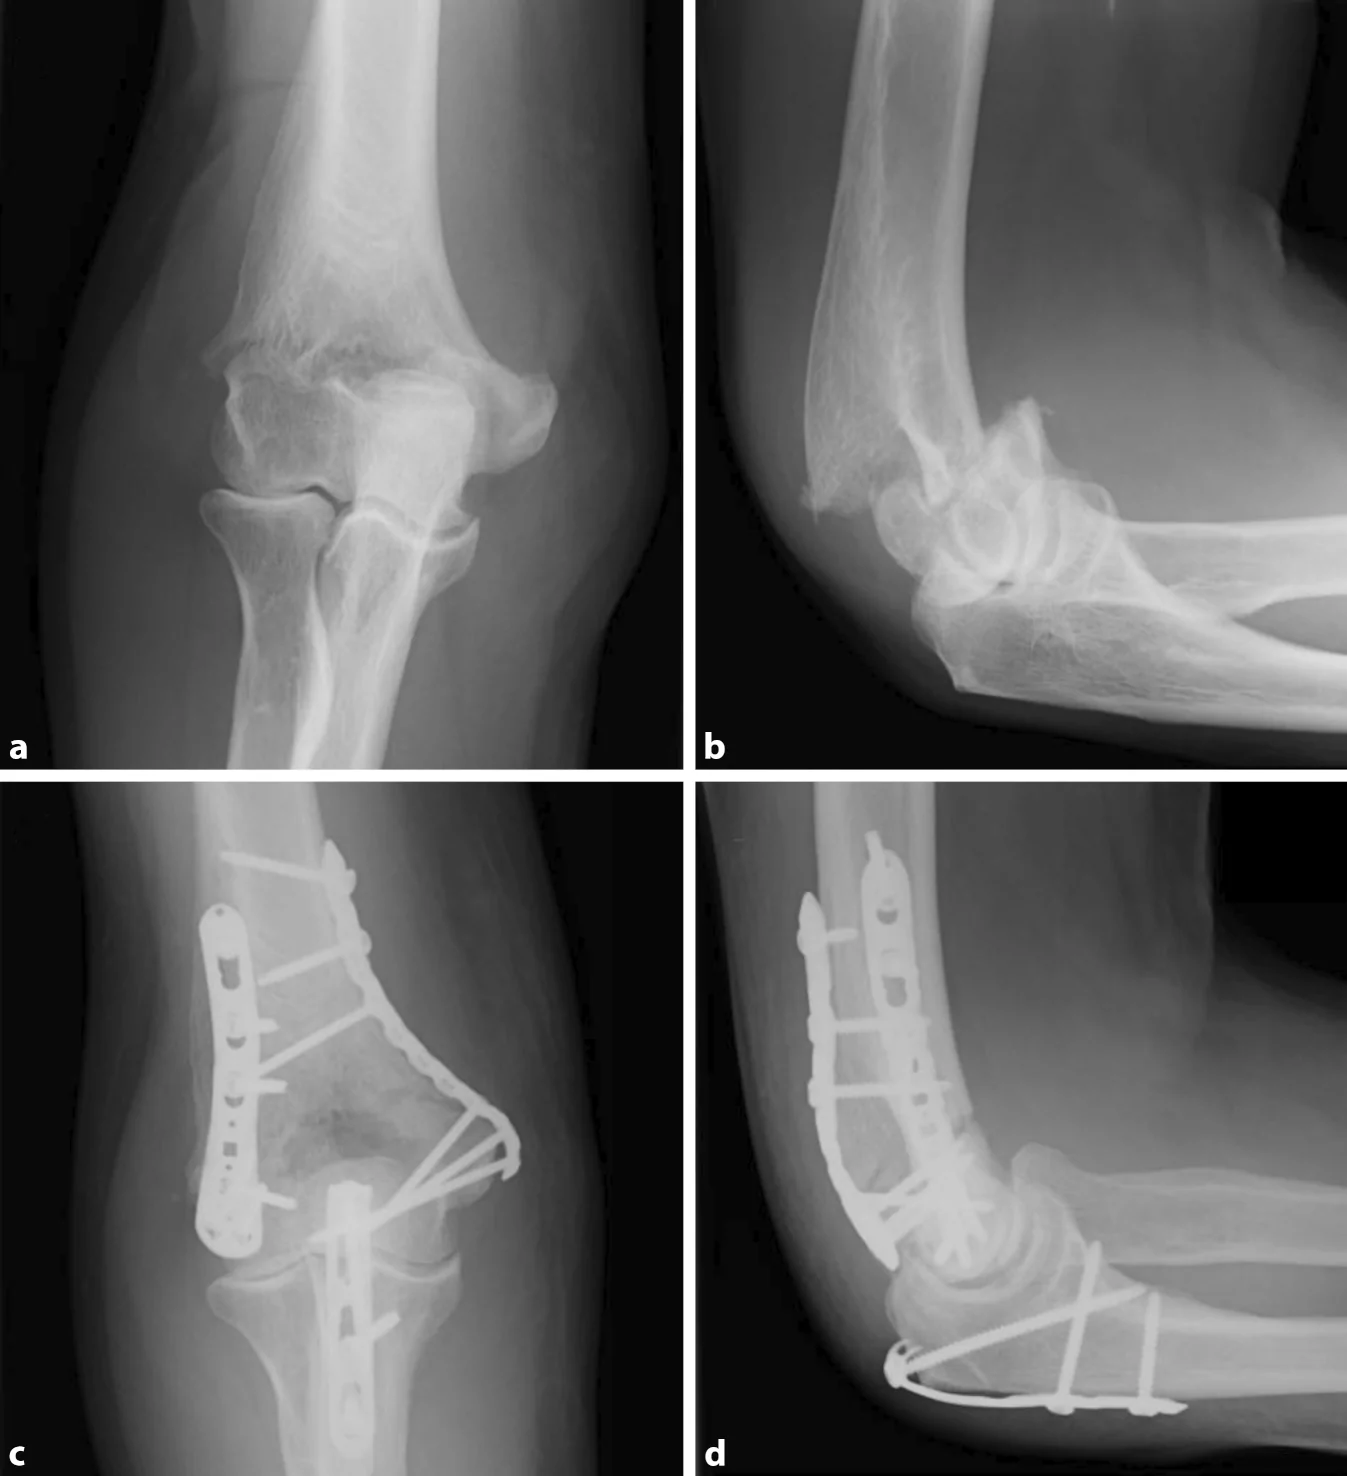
A 68-year-old man sustained an extra-articular fracture of the distal humerus treated with double plating using the flip osteotomy approach. The osteotomy was secured with a plate. a, b Preoperative images of the extra-articular fracture. c, d X-rays obtained at the 6‑week follow-up
